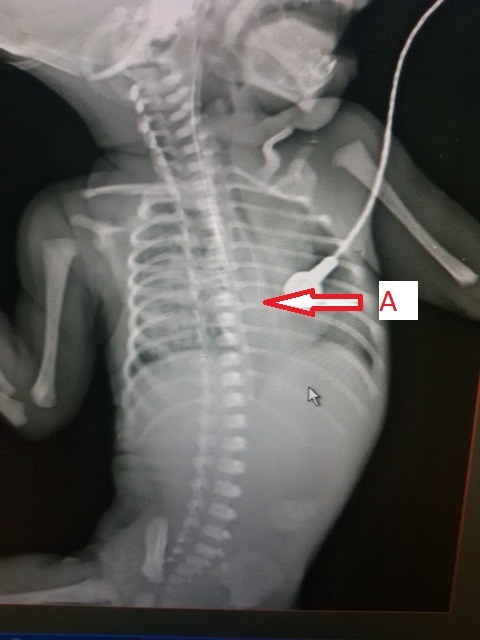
Figure 1: The X-ray with nasogastric tube in situ showing the level of upper pouch at the level of T7–T8 vertebrae (A) and gasless abdomen
